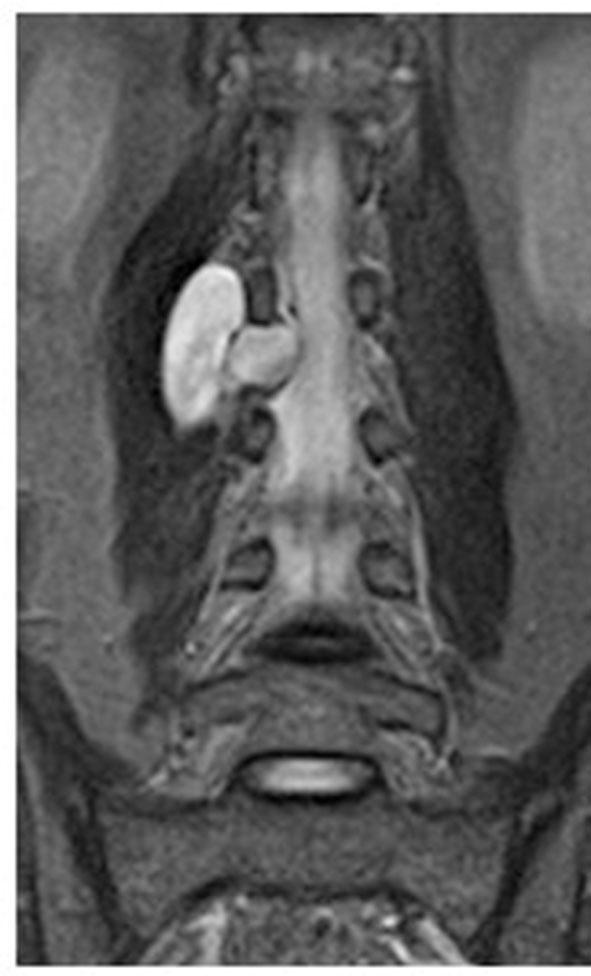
Preoperative contrast-enhanced MRI of the lumbar spine.
